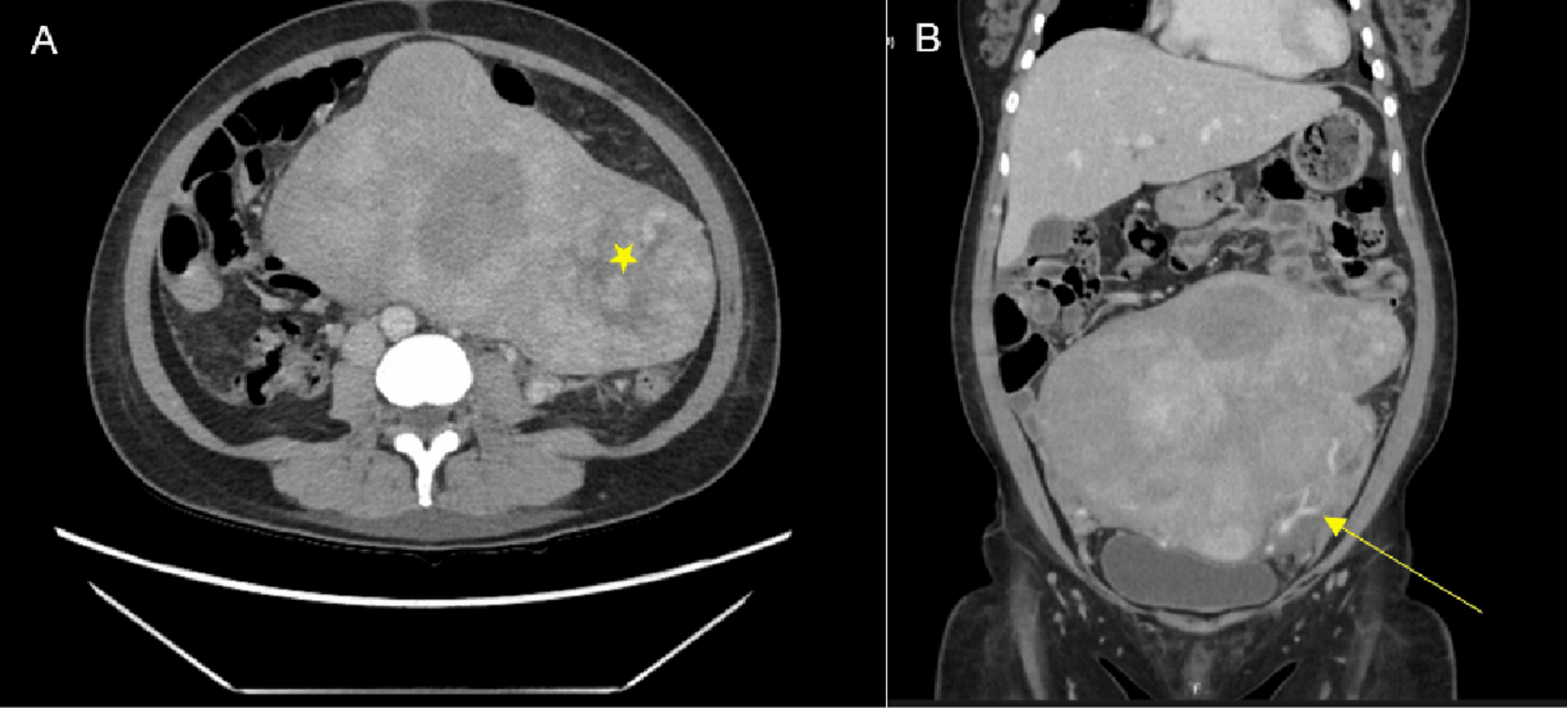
Computed tomography of the abdomen (A axial, B coronal) demonstrating giant fibroids (*) and colonic stranding (arrow) of diverticulitis.
